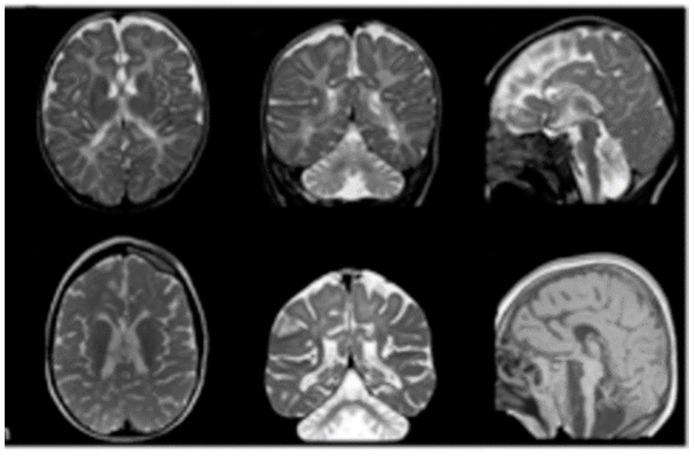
Magnetic resonance imaging. Images are 1.5 to 3 T and include T1-weighted, T2-weighted and fluid-attenuated inversion recovery (FLAIR) sequences. Structural abnormalities include cerebellar and brainstem atrophy, dilated ventricles and subarachnoid spaces, thinning of the corpus callosum and hypomyelination.
